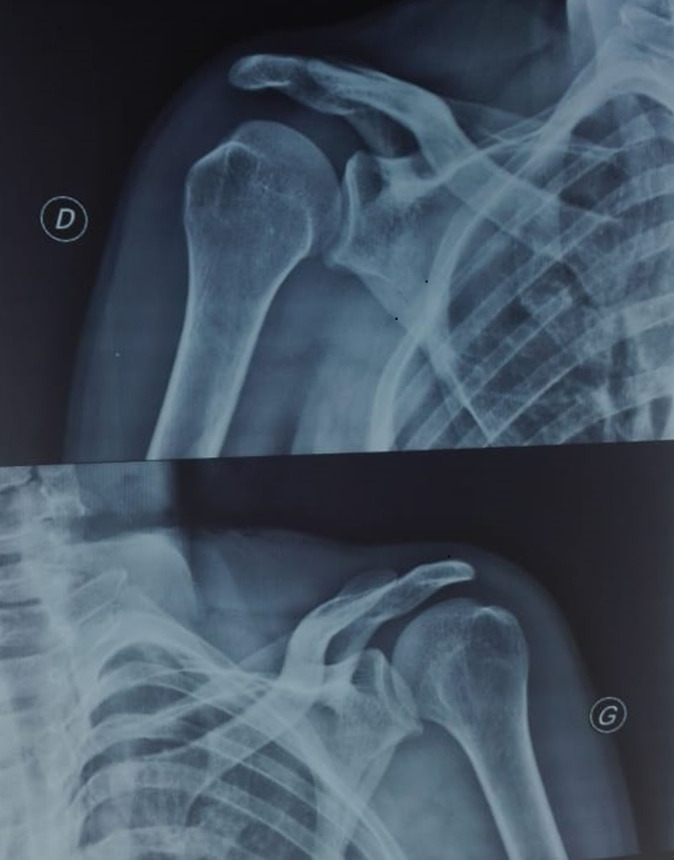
control X-ray after reduction of shoulders dislocation
